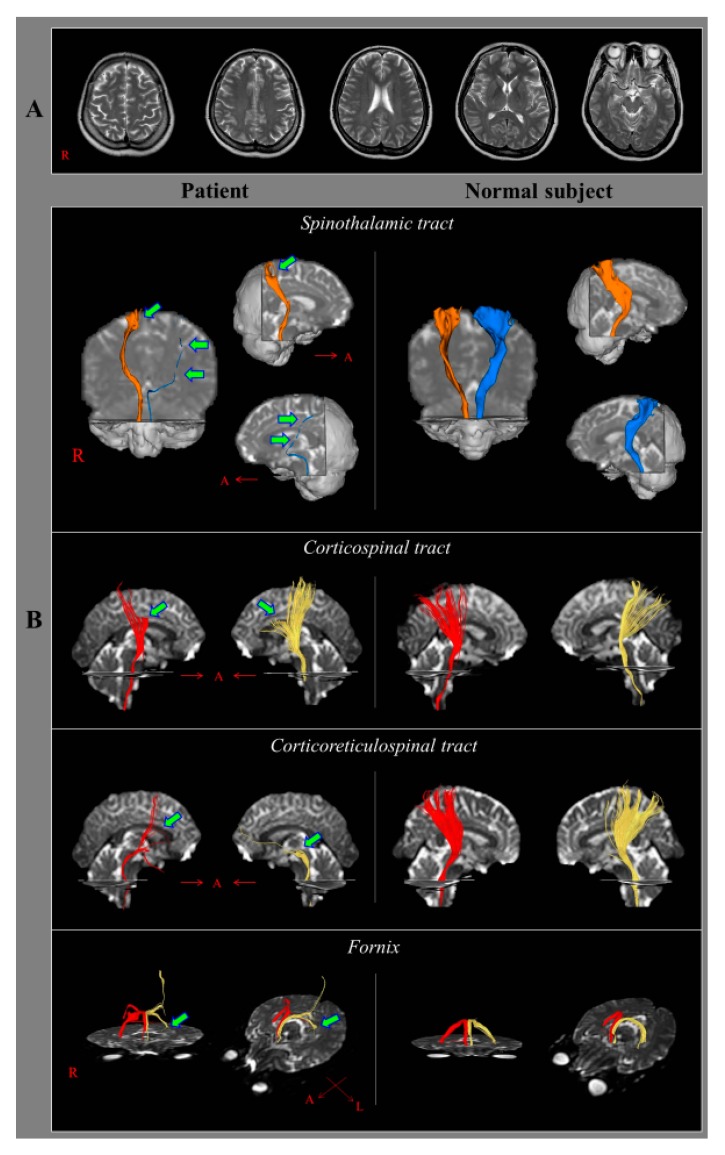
(A) T2-weighted brain magnetic resonance images at nine years after whiplash injury onset shows no abnormality. (B) Results of diffusion tensor tractography of the patient at nine years after injury compared to those from a normal subject (42-year-old female). The left spinothalamic tract shows marked narrowing (green arrows) while the right spinothalamic tract reveals mild narrowing and partial tearing in the upper portion (green arrow). In addition, partial tears at the subcortical white matter (green arrows) are visible in both corticospinal tracts and the right corticoreticulospinal tract. Discontinuations (green arrows) are observed in the left corticoreticulospinal tract and the left fornical crus. Orange color—right spinothalamic tract; blue color—left spinothalamic tract; red color—right corticospinal tract, corticoreticulospinal tract, fornix, yellow color—left corticospinal tract, corticoreticulospinal tract, fornix; A: anterior, R: right, L: left.
